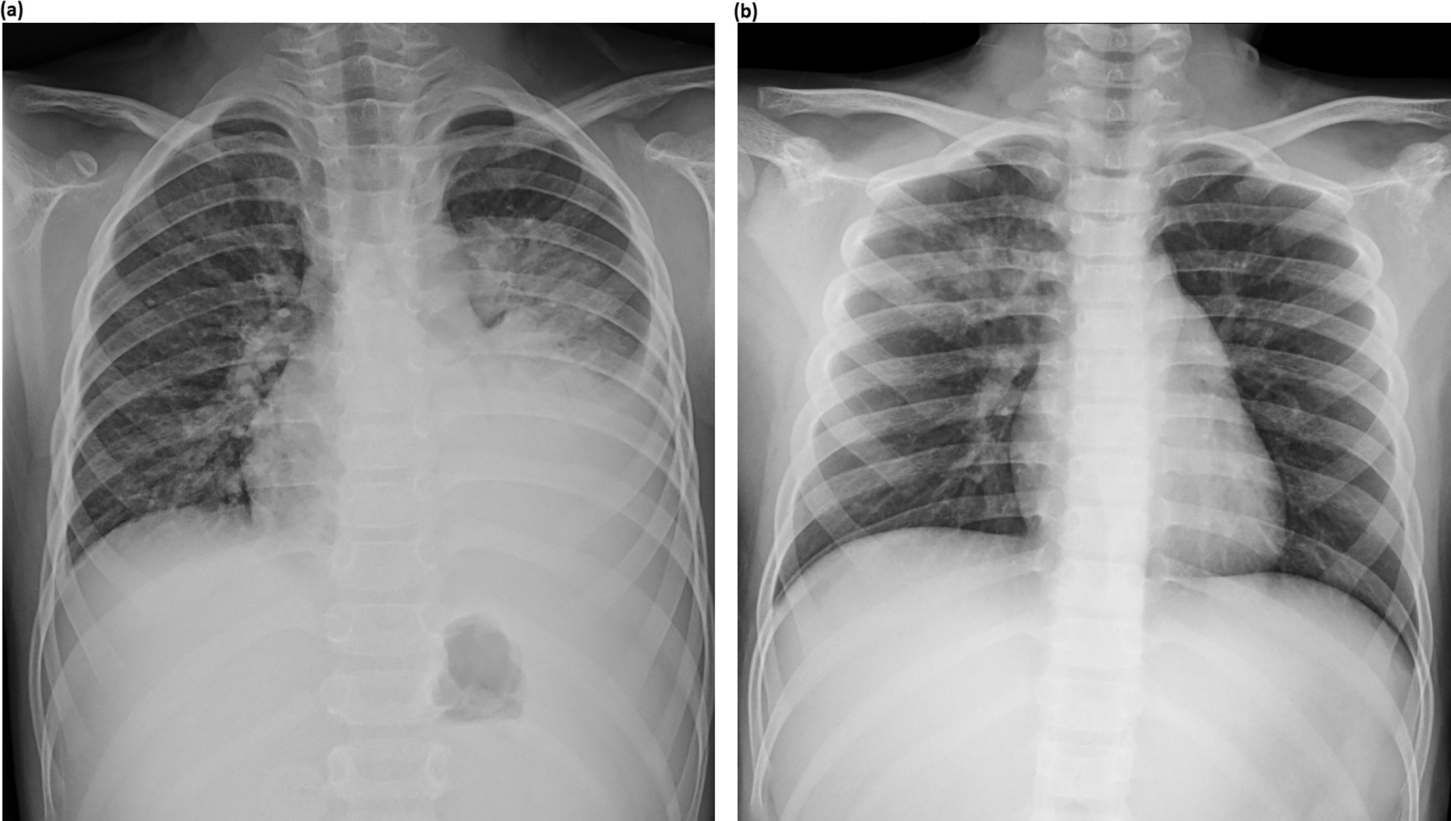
Determination of the dominant radiographic finding. (a) A 15-year-old boy with *Mycoplasma pneumoniae* pneumonia. Chest radiograph shows lobar consolidation in the left lower lobe and parahilar peribronchial infiltration in the left lung. In this case, lobar consolidation was regarded as the dominant finding, and categorized as *lobar or segmental consolidation*. (b) Chest radiograph of a 9-year-old girl with *Mycoplasma pneumoniae* pneumonia shows both a localized reticulonodular opacity and a patchy opacity in the right upper lung. In this case, the focal reticulonodular opacity was regarded as the dominant finding, and categorized as *localized reticulonodular infiltration*.
